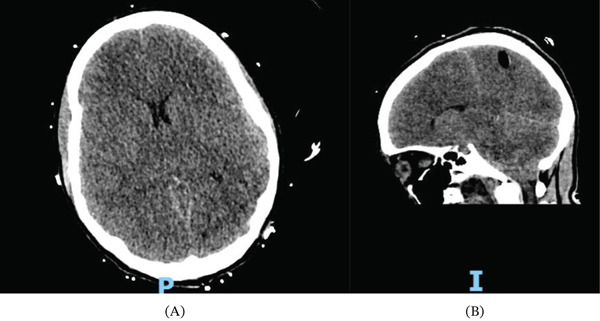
Cerebral CT imaging after the disclosure of bilateral areflexic mydriasis showing pneumocephalus and diffused cerebral edema. (A) Axial view and (B) coronal view (P: posterior; I: inferior).
